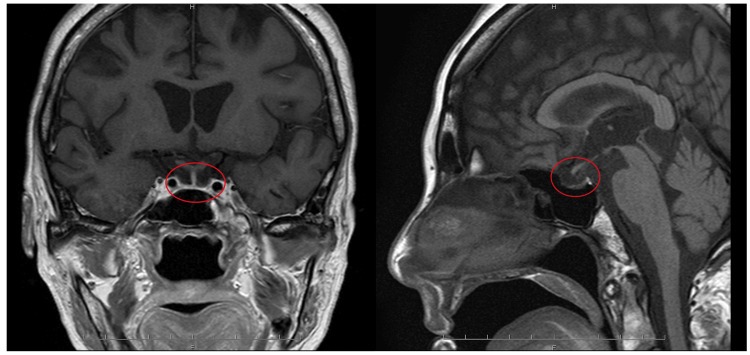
Pituitary MRI taken on day 28 (T1WI) Left panel: Coronal gadolinium-enhanced T1WI. Right panel: Sagittal non-contrast T1WI. The structures within the red circles represent the pituitary gland. Both images demonstrate a flattened pituitary gland compressed against the floor of the sella turcica, consistent with an empty sella. The posterior pituitary bright spot is preserved, and there is no deviation of the pituitary stalk. T1WI: T1-weighted imaging
